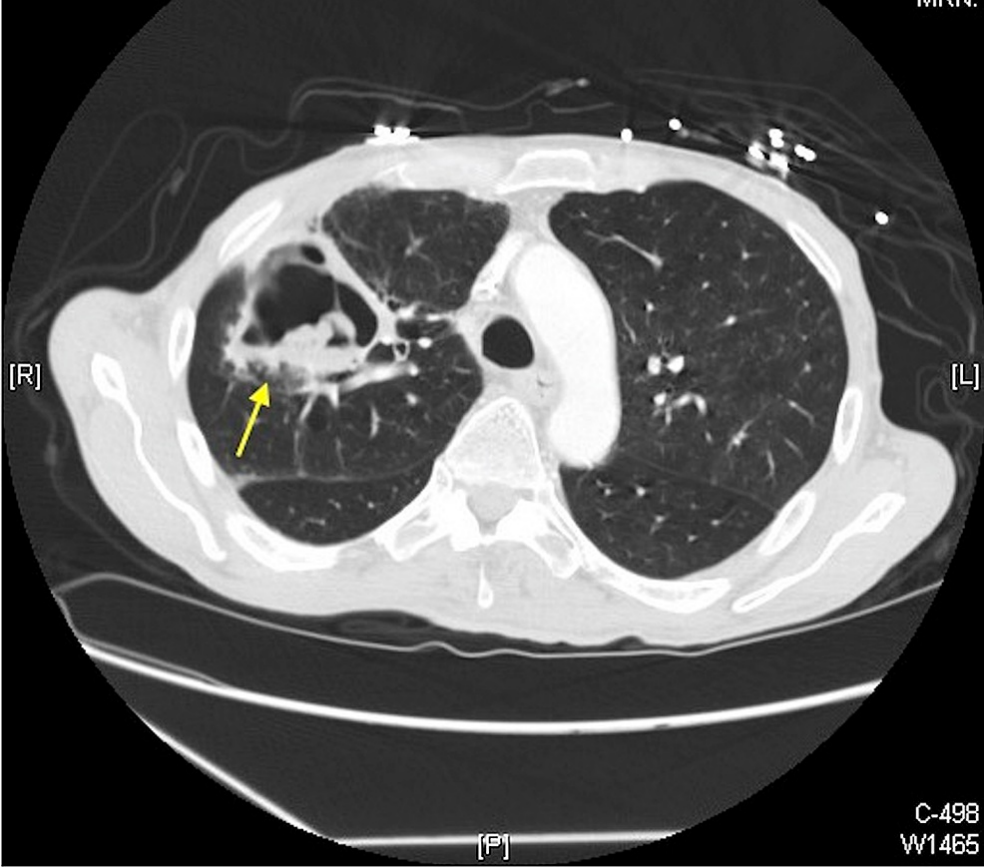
Axial reformatted CT chest with IV contrast demonstrating a cavitary lesion with internal dependent debris and an air-fluid level in the right upper lobe apical and anterior segment measuring 5.7 × 5.7 × 5.8 cm (yellow arrow). CT: computed tomography; IV: intravenous
